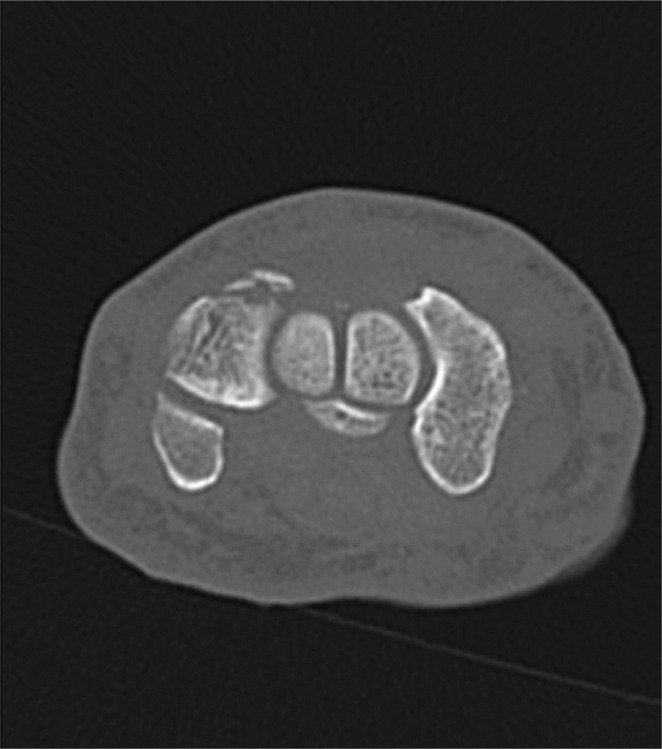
Typical CT scan finding in axial reconstruction
